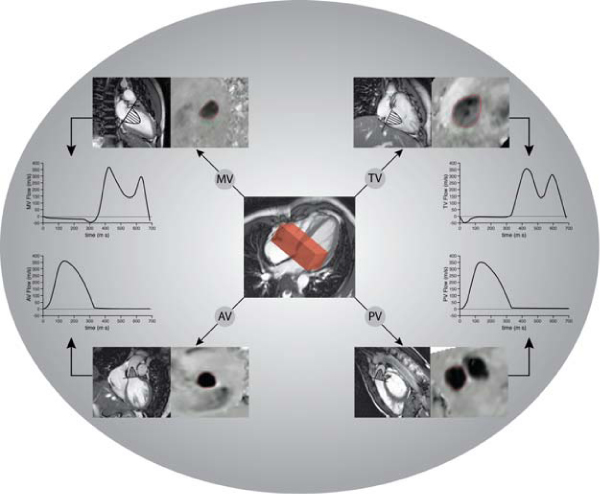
3D 3-directional velocity-encoded MRI is performed at the basal level of the heart. In offline analysis, retrospective valve tracking for each of the heart valves can be performed, resulting in flow at the mitral valve (MV), tricuspid valve (TV), aortic valve (AV) and pulmonary valve (PV).
